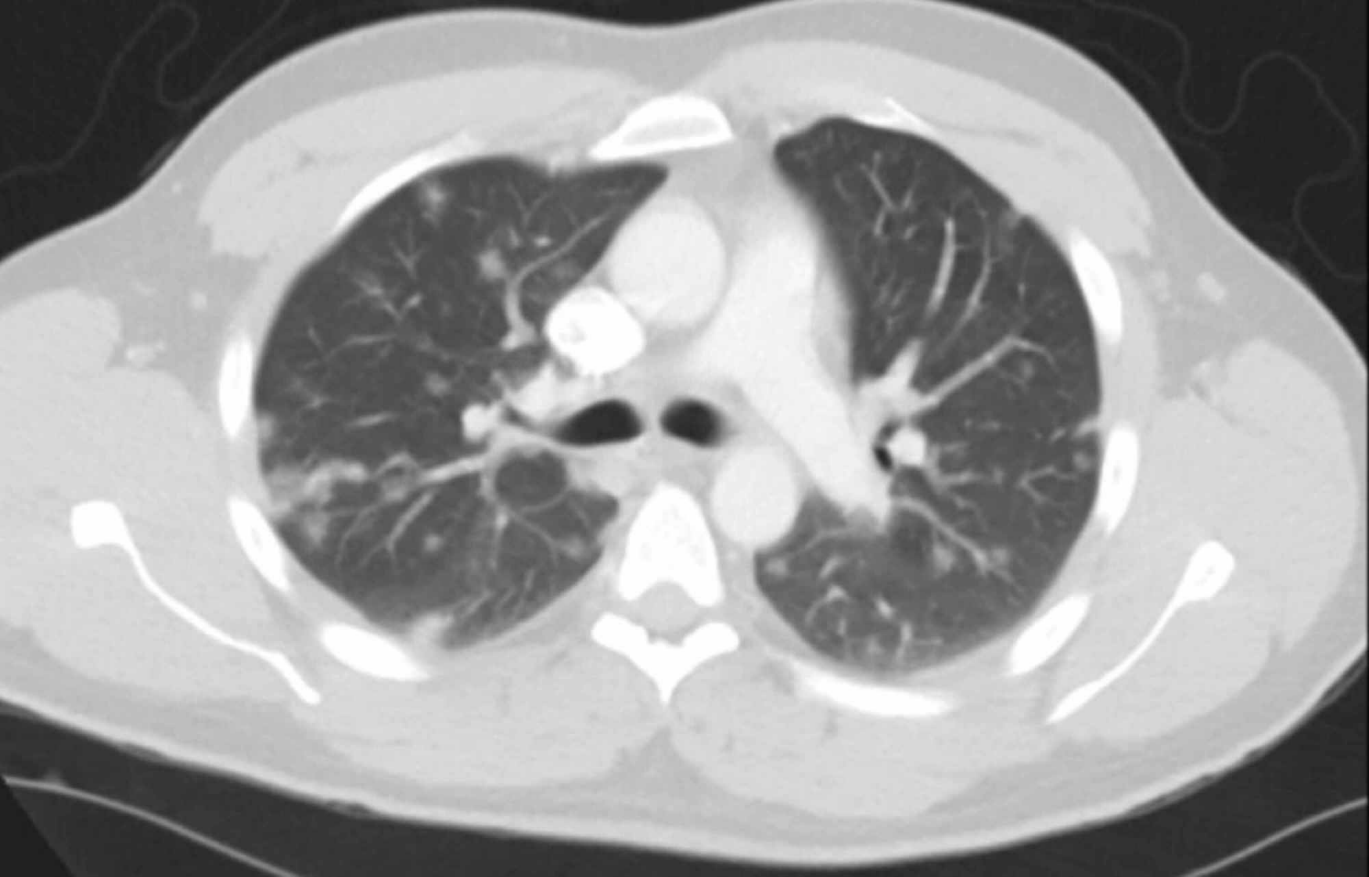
Axial computed tomography (CT) image of the chest showing bilateral ground-glass opacities
